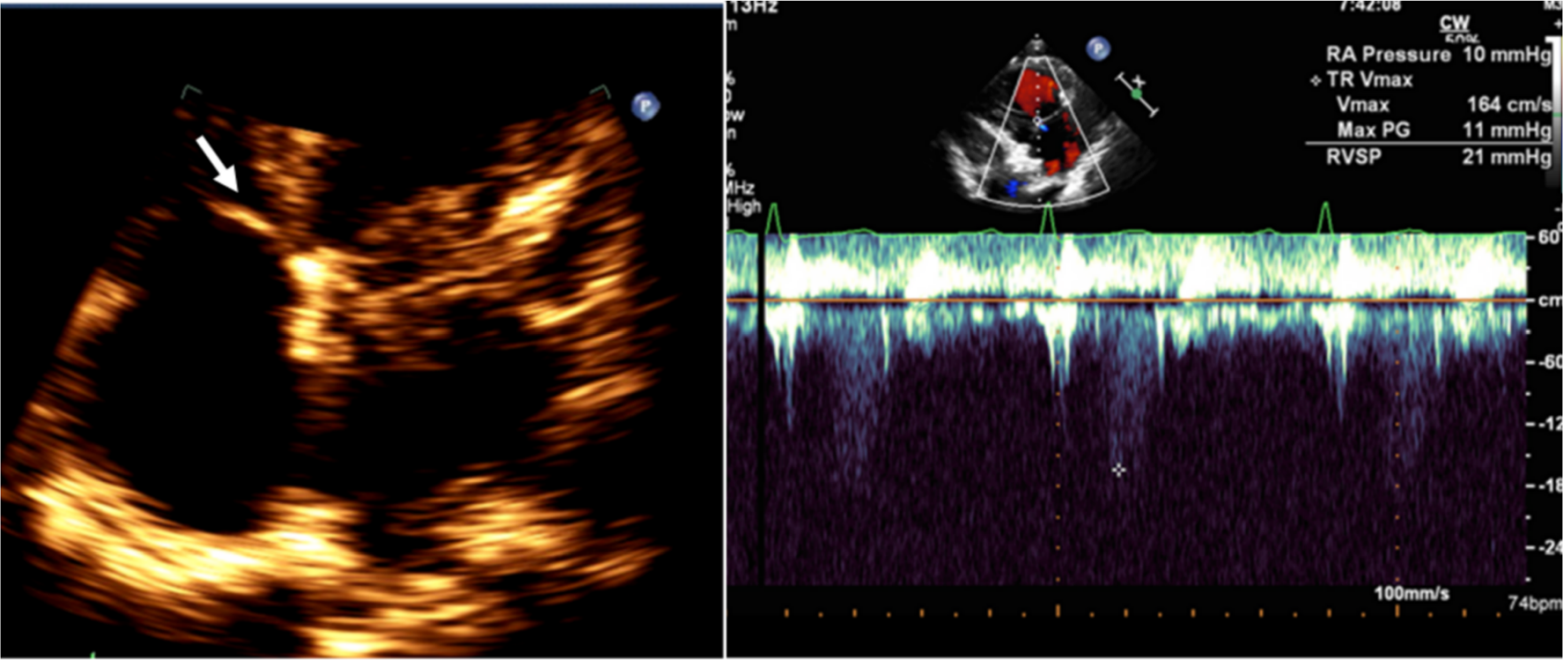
Normal echocardiographic appearance of tricuspid valve (*arrow*) and wave pattern
